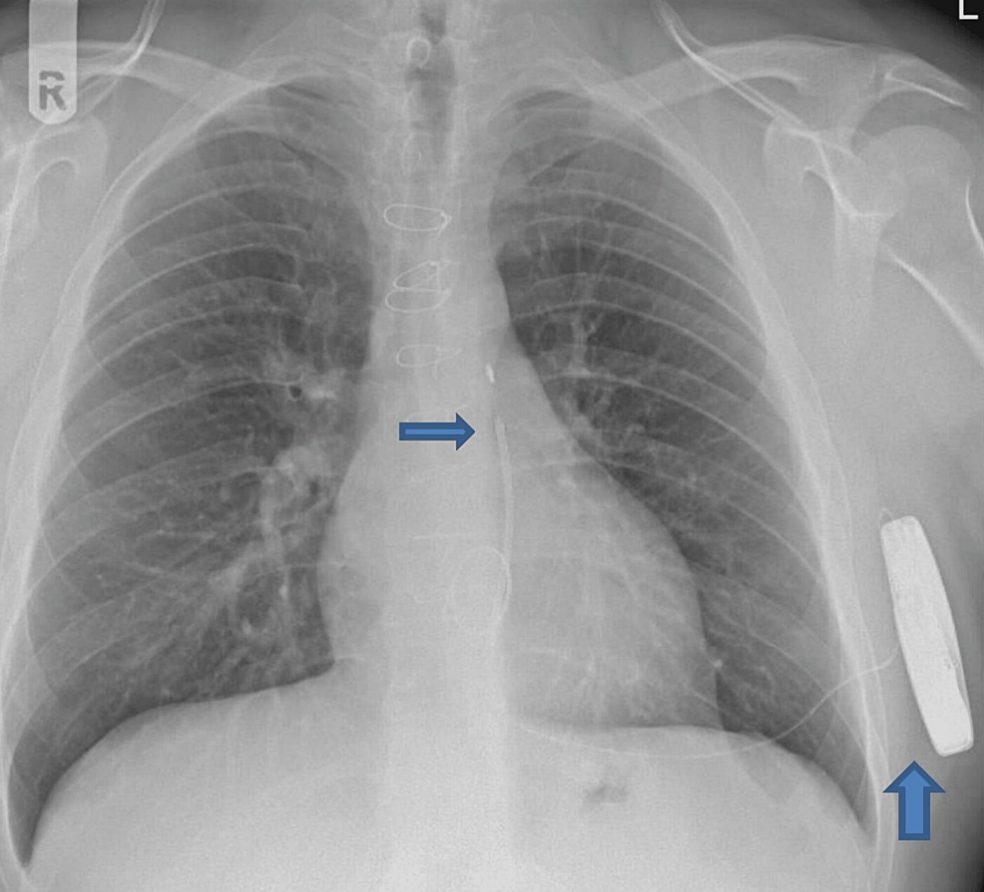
Chest radiography AP view showing the ICD in situ (pointed arrow). ICD = implantable cardioverter defibrillator; AP = anteroposterior view
